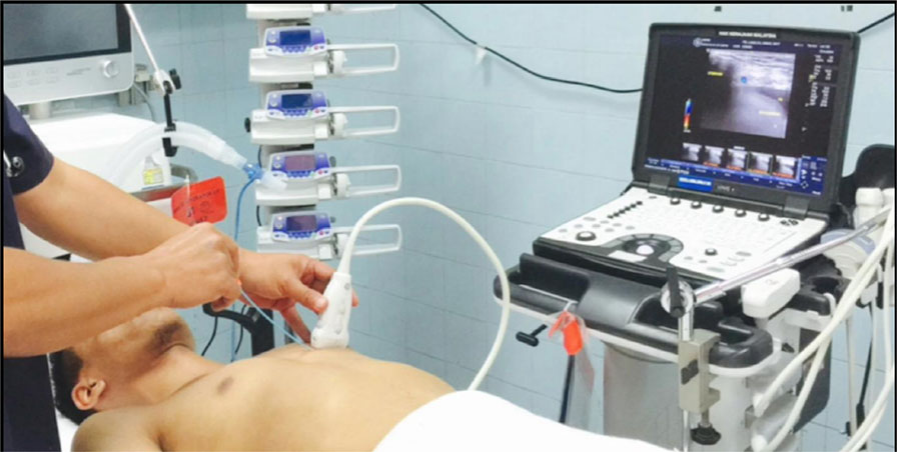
Parasternal approach with needle directed from medial to lateral technique.
